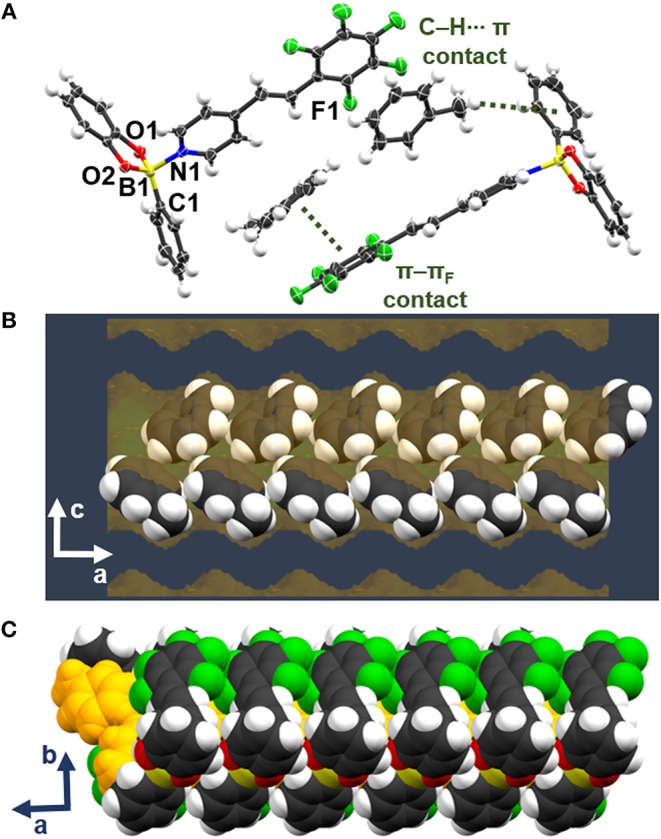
X-ray structure be-pf-sbz⊃tol: (A) stacking involving T-shaped adducts be-pf-sbz⊃tol, (B) corrugated tol stacks along the *a*-axis, and (C) space-filling view of channel confinement.
